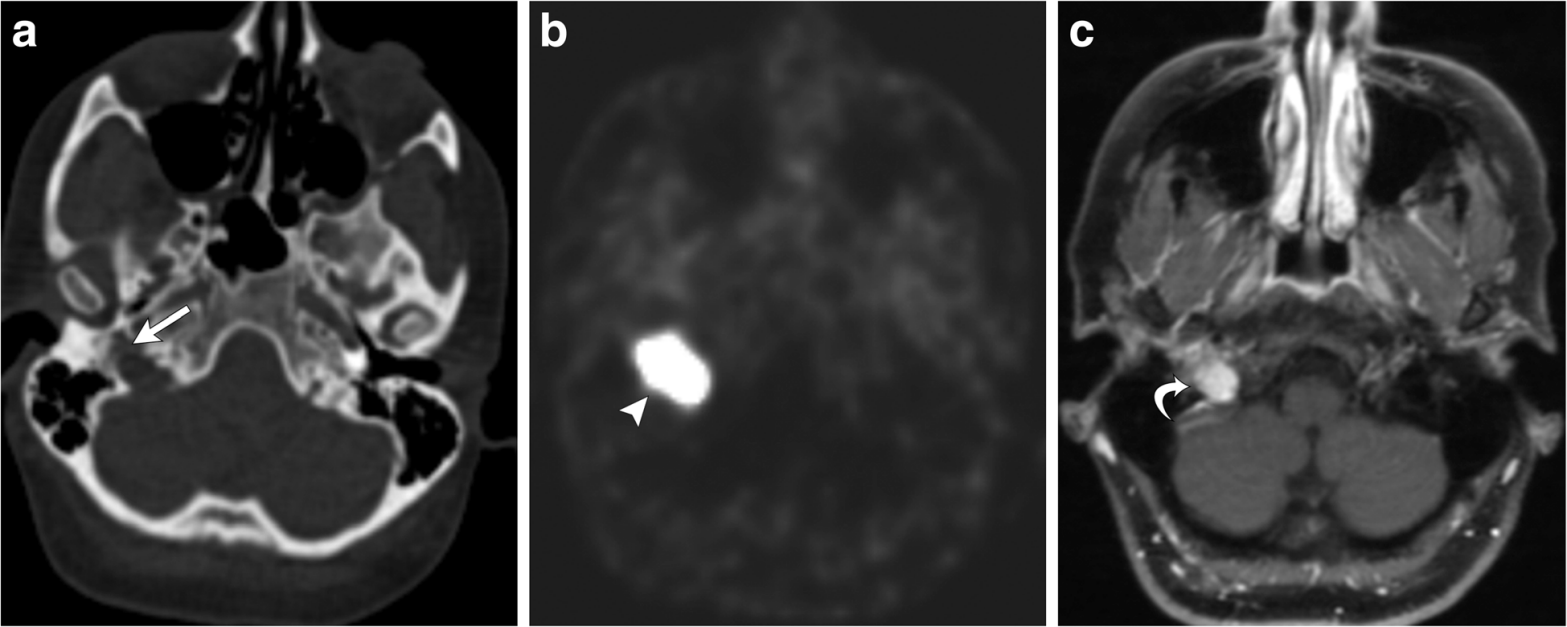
Axial CT (a) demonstrates widening right jugular bulb, with a moth-eaten appearance of the walls of the right jugular foramen (white arrow). Axial attenuation-corrected PET (b) demonstrates marked FDG uptake within the jugular foramen consistent with hypermetabolic activity (white arrowhead). T1-weighted post-contrast MR (c) demonstrates a well-defined, enhancing, expansile mass centered in the right jugular foramen (curved white arrow)
